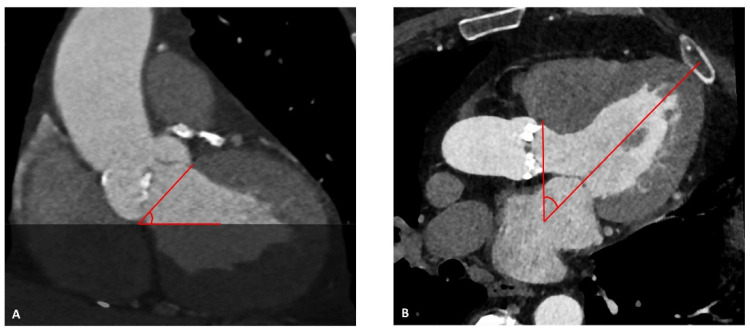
Assessment of aortic and annular angle. Pre-TAVI CTA reconstructions for measurement of the aortic angle between the annular and ideal horizontal plane (A) and the annular angle between the annular plane and the longitudinal axis of the left ventricle (B).
